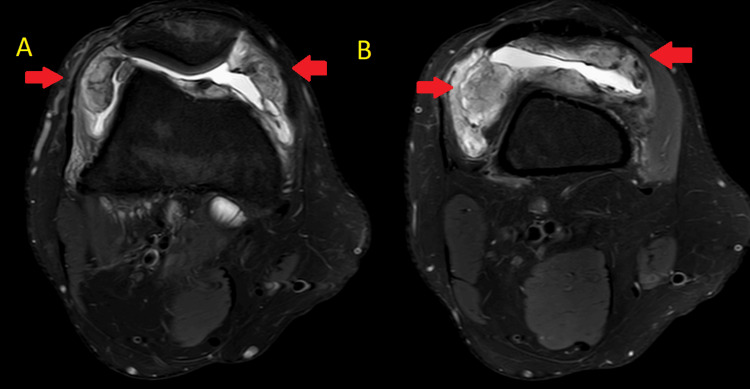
Axial MRI sections (A) and (B) showing multiple loose bodies T2-weighted images (A) and (B) show multiple loose bodies around the knee MRI: magnetic resonance imaging
